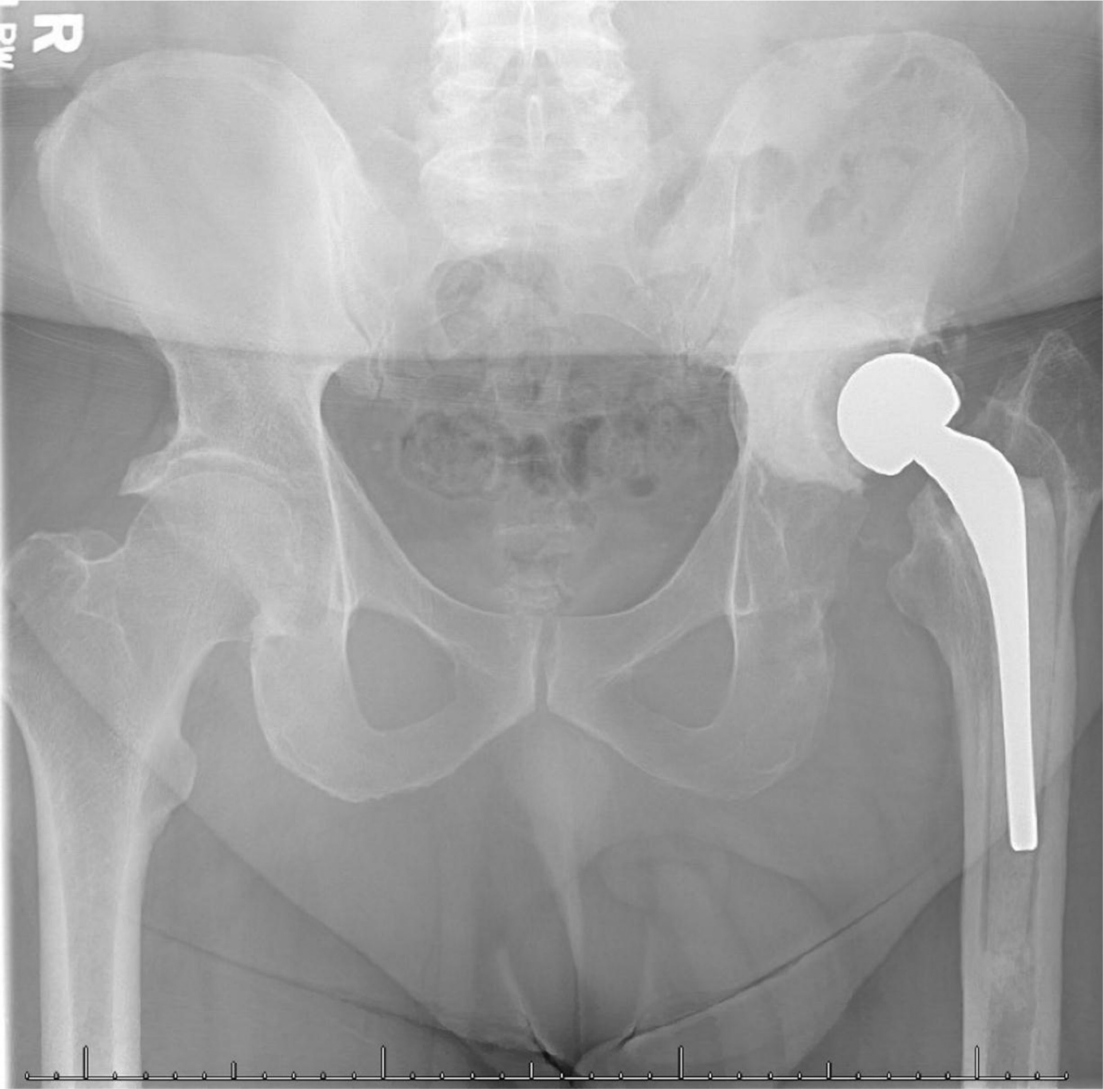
Anteroposterior radiograph of the pelvis showing a Prostalac spacer retained for 11 years.
